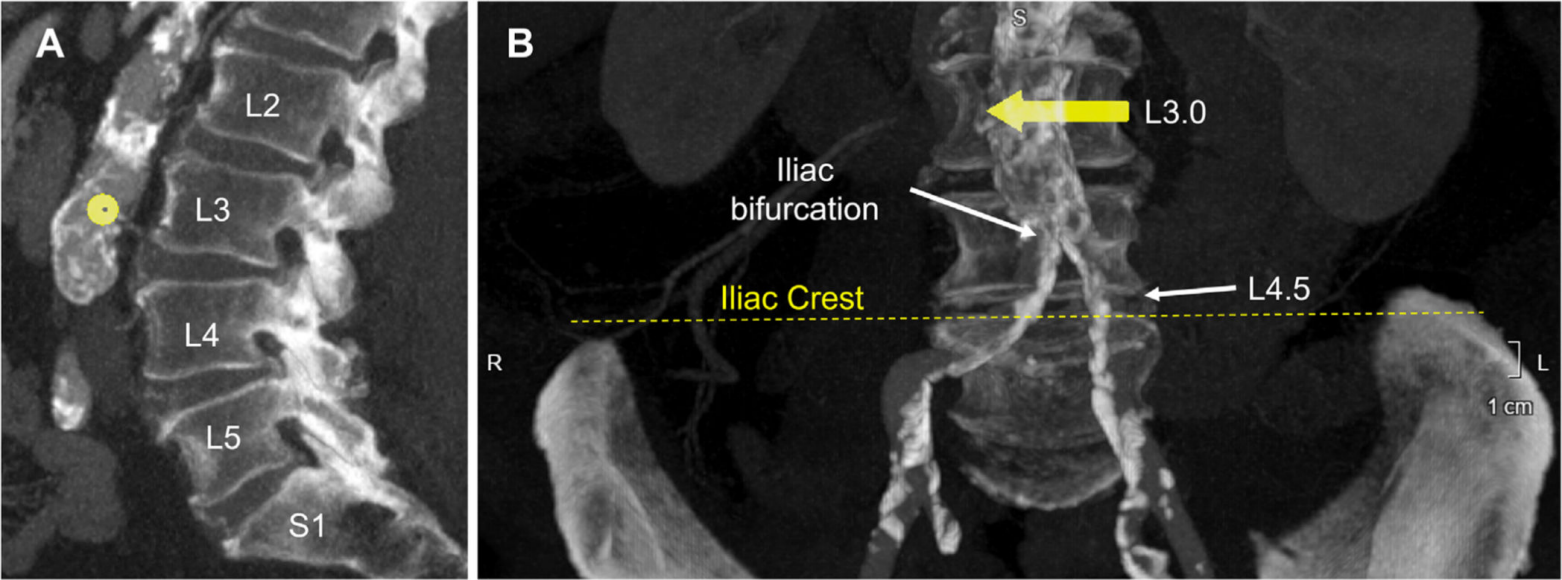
Landmarks Landmarks to coregister computed tomography–based traversal targets with fluoroscopy. (A) Lumbar vertebrae on sagittal reconstruction. The trapezoidal S1 sacral vertebra is distinguished from the rectangular L5 vertebra. (B) Iliac crests correspond with L4-L5 vertebral interspace and aortoiliac bifurcation. This target overlies the mid L3 (L3.0) vertebra.
